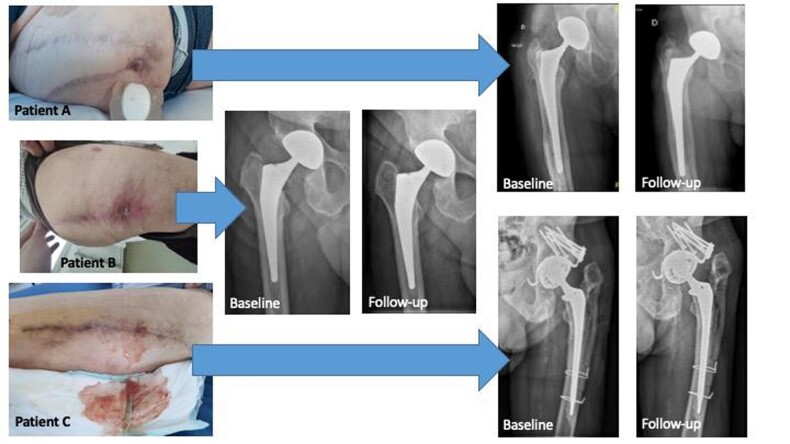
Local signs of infection with discharge of the three patients (A, B and C) with complex relapsing prosthetic hip infection with X-ray before the LysinDAIR procedure and at 2 years of follow-up
